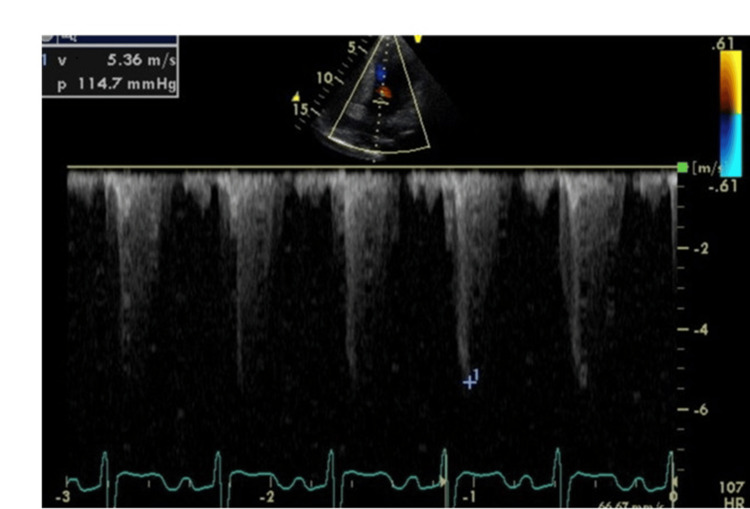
Echocardiography at 24 weeks of the first pregnancy The left ventricular outflow tract pressure gradient worsened to 114 mmHg.
